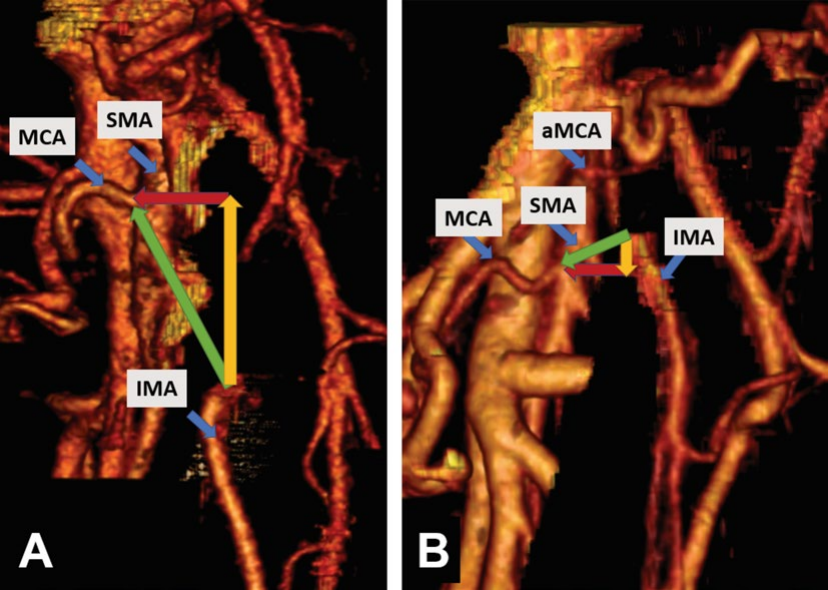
A CT reconstruction visualizing the mesenteric inter-arterial stair in two patients. A An MCA origin cranial to the IMA origin implies a positive Distance B (yellow) and that Distance A (green) points from the IMA origin cranioventrally towards the MCA origin. Distance C (red) represents the height of the stair. In this patient Distance A was 4.23 cm, Distance B 4.07 cm, Distance C 1.16 cm and the angle between Distance A and B 15.9 degrees. B An MCA origin caudal to the IMA origin implies a negative Distance B (yellow) and that Distance A (green) points caudoventrally. In this patient Distance A was 2.34 cm, Distance B − 0.58 cm, Distance C 2.26 cm and the angle between Distance A and B 75.6 degrees Vessels *aMCA* accessory middle colic artery, *MCA* middle colic artery, *SMA* superior mesenteric artery, *IMA* inferior mesenteric artery (Color figure online)
